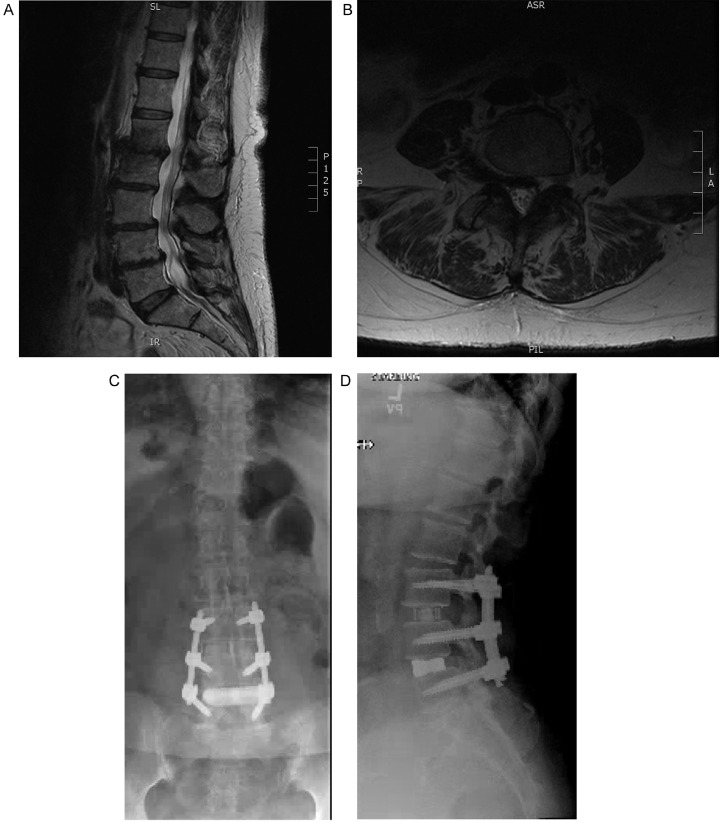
A. pre-operative sagittal T2 MRI of the lumbosacral spine with an L4-5 spondylolisthesis and degenerative disc disease at multiple levels in the lumbar spine, including grade four facet joint deterioration at L4-5 with locked facets. B: pre-operative axial T2 MRI of the lumbosacral spine with an L4-5 spondylolisthesis and degenerative disc disease at multiple levels in the lumbar spine, including grade four facet joint deterioration at L4-5 with locked facets. C: post-operative AP lumbosacral X-ray demonstrating interval placement of interbody cage and posterior instrumented fixation. D: post-operative lateral lumbosacral X-ray demonstrating multilevel interbody cage placement and pedicle screw fixation with maintained alignment of the lumbar spine.
